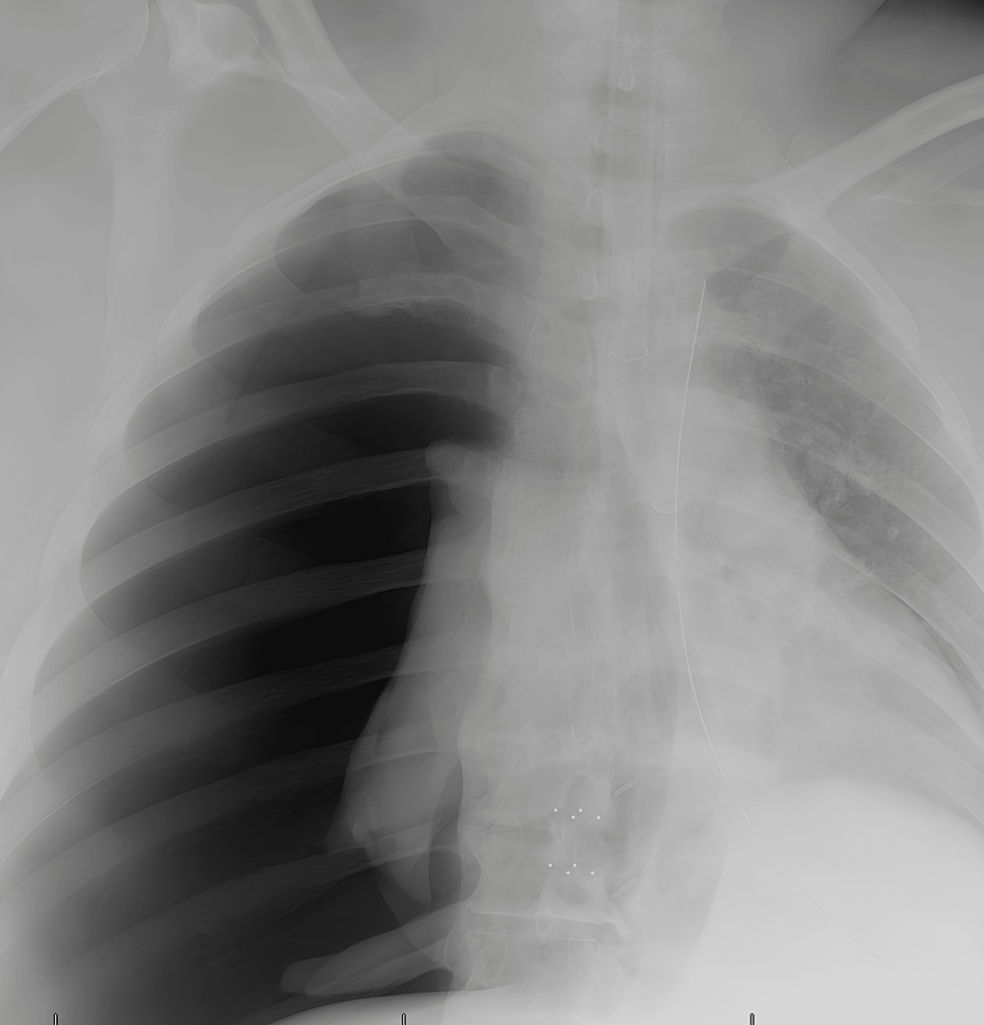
Right lung was completely compressed on bedside chest x-ray.
